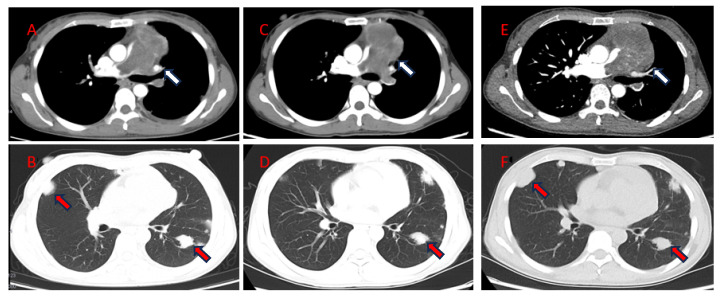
The second CT examinations (A,B) (12 May 2022), the third CT examinations (C,D) (3 July 2022), and the fourth CT examinations (E,F) (26 July 2022) showed that the volume of soft tissue masses in the anterior mediastinum and pulmonary artery had not changed (white arrow) and the volume of metastatic tumors in both lungs had decreased during chemotherapy (red arrow). CT, computed tomography.
